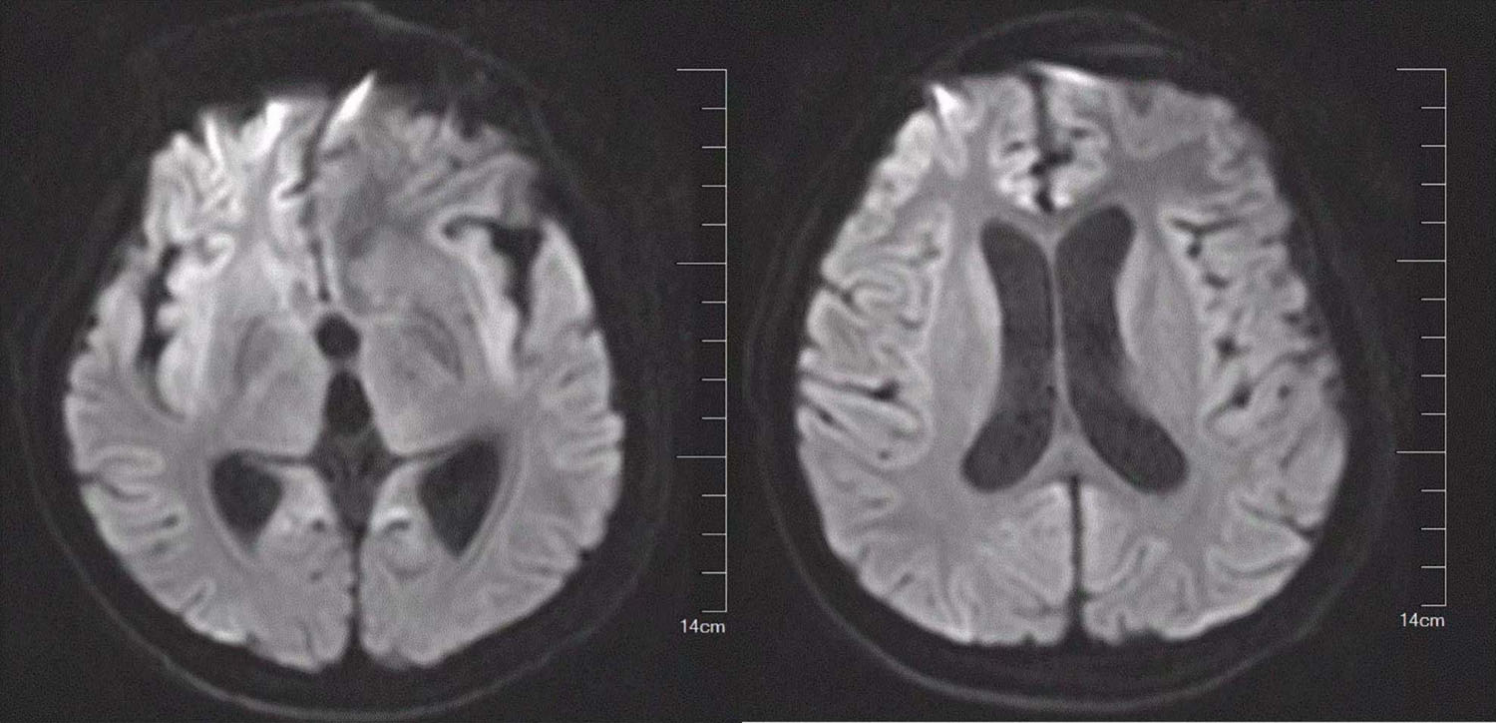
Preoperative cranial MRI
